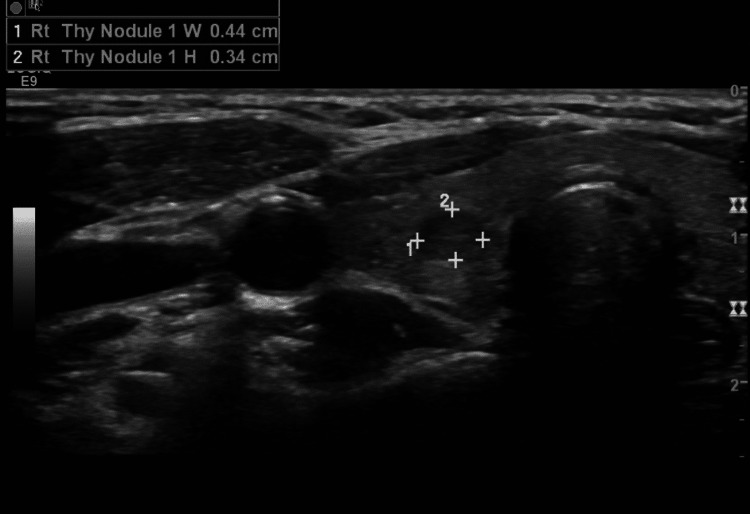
Ultrasound of the patient four years after the initial presentation The image shows a hypoechoic nodule in the right thyroid lobe, measuring 0.4 x 0.3 cm Rt: right. Thy: thyroid. W: width. H: height
